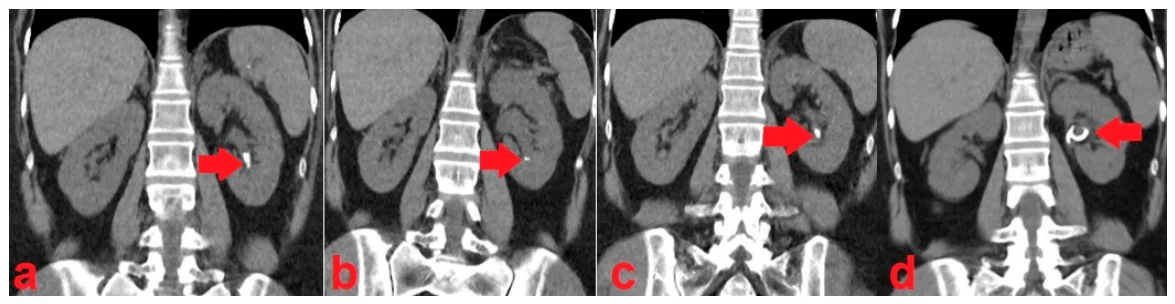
Serial non-contrast computed tomography (NCCT) images of a patient requiring secondary retrograde intrarenal surgery (RIRS): (a) Preoperative NCCT demonstrating an isolated lower-pole calculus (arrow); (b) Baseline NCCT obtained at 3 months postoperatively establishing eligibility with a 4.0 mm residual stone fragment; (c) Follow-up NCCT at 39 months postoperatively revealing interval stone enlargement to 9.0 mm (arrow); and (d) Control NCCT confirming complete stone clearance following secondary RIRS at 42 months postoperatively (arrow: JJ stent) (terminal stone burden: 0.0 mm^2^).
